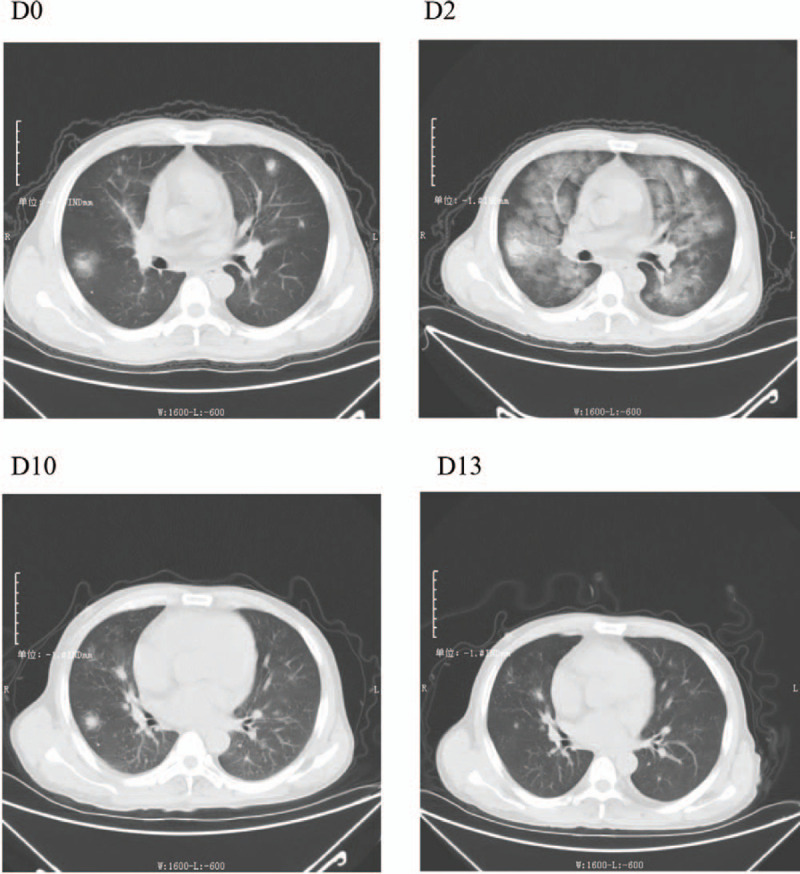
The changes in the lung plain CT scan of case 1 throughout treatment. D0. Bilateral lung inflammation. D2. Bilateral lung lesions were advanced. D10. Bilateral lung lesions were decreased. D13. The lesion range was reduced, and the density was light.
